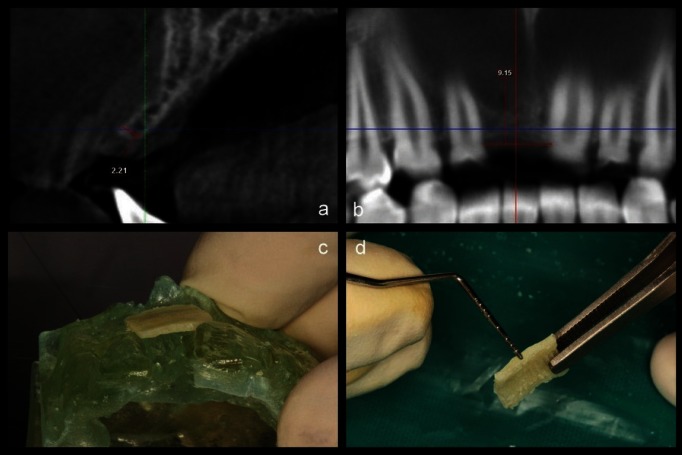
a) Pre-operative radiographic (saggital) view of ridge width. b) Pre-operative radiographic (panoramic) view of ridge width. c) 3-D stereolithographic model of the recipient site. d) Cortical lamina trimmed to appropriate dimensions.
